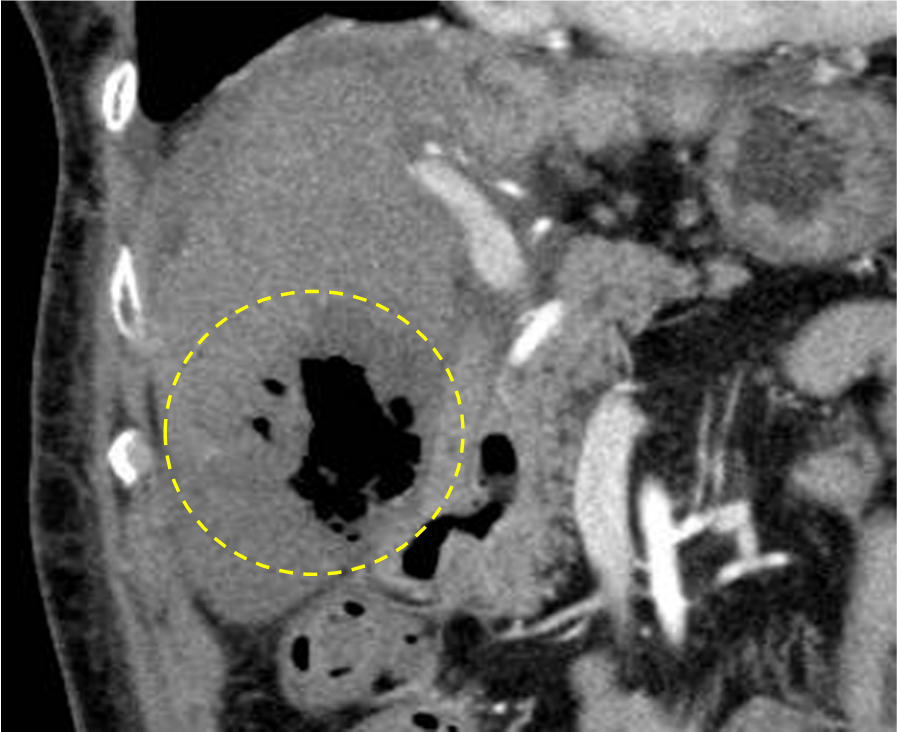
Contrast-enhanced computed tomography findings on POD 54. A liver abscess 70 mm in size was found in segment 6. There was no intrahepatic bile duct dilatation or ischemic areas in the remnant liver
